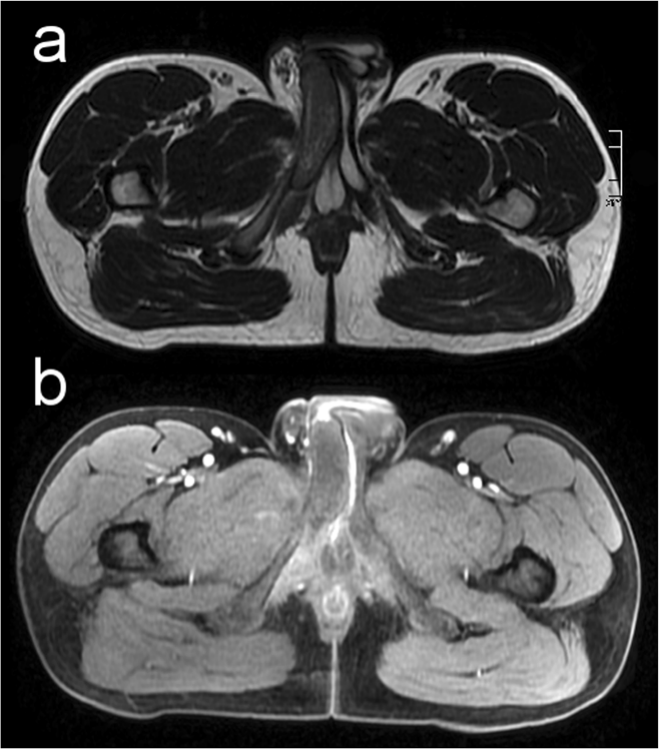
Transversal T2-weighted (a) and fat saturated T1-weighted (b) MRI images through the basis of the penis. Arrows show thrombosis of the right proximal corpus cavernosum.
